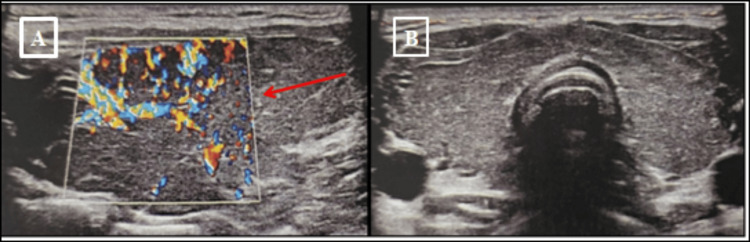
Thyroid ultrasound showing a hypoechoic and inhomogeneous gland with increased vascularity, as demonstrated by color Doppler imaging on the left (highlighted by the red arrow), and a transverse view on the right confirming features of autoimmune thyroiditis. (A) Longitudinal Doppler ultrasound of the thyroid showing increased intraparenchymal vascularity (highlighted by the red arrow), a typical feature of autoimmune thyroiditis; (B) Transverse grayscale ultrasound image of the thyroid gland confirming diffuse hypoechoic and heterogeneous echotexture, consistent with autoimmune involvement.
